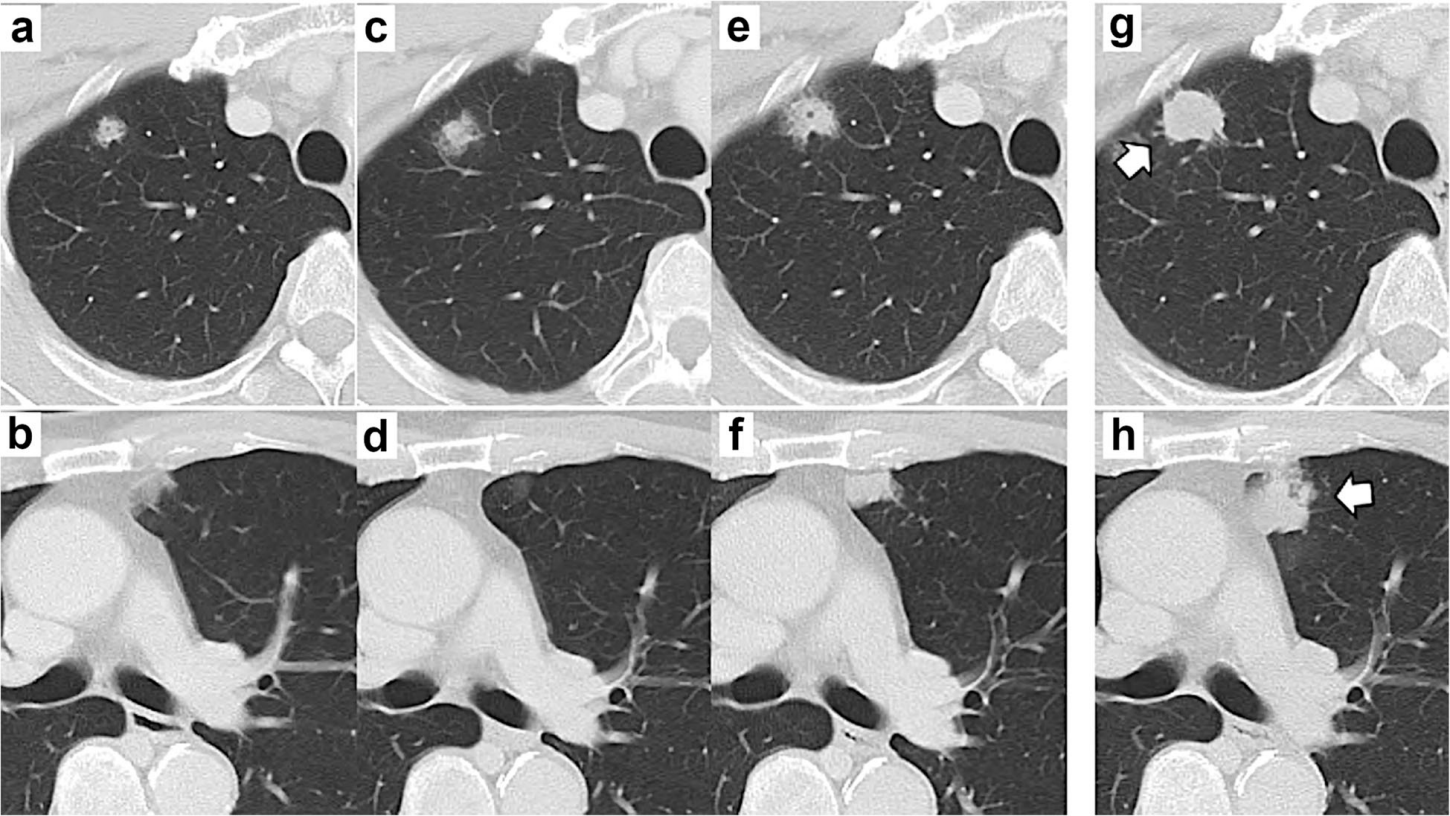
Imaging findings for both of the lung metastases. Computed tomography showed a 5-mm-sized frosted glass shadow in the right lung apex (a), and a 5-mm-sized node from the left superior mediastinum to the ventral pleura in the left lung (b) 42 months after first resection. Forty-six months after first resection, the right lesion changed to a nodule and expanded to 10 mm (c), the left lesion disappeared (d). Both the right (e) and left lesions (f) indicated growth again 50 months after first resection. The size of both tumors, the right (g: white arrow) and left lesion (h: white arrow) had increased to 20 mm right before resection 54 months after first resection
